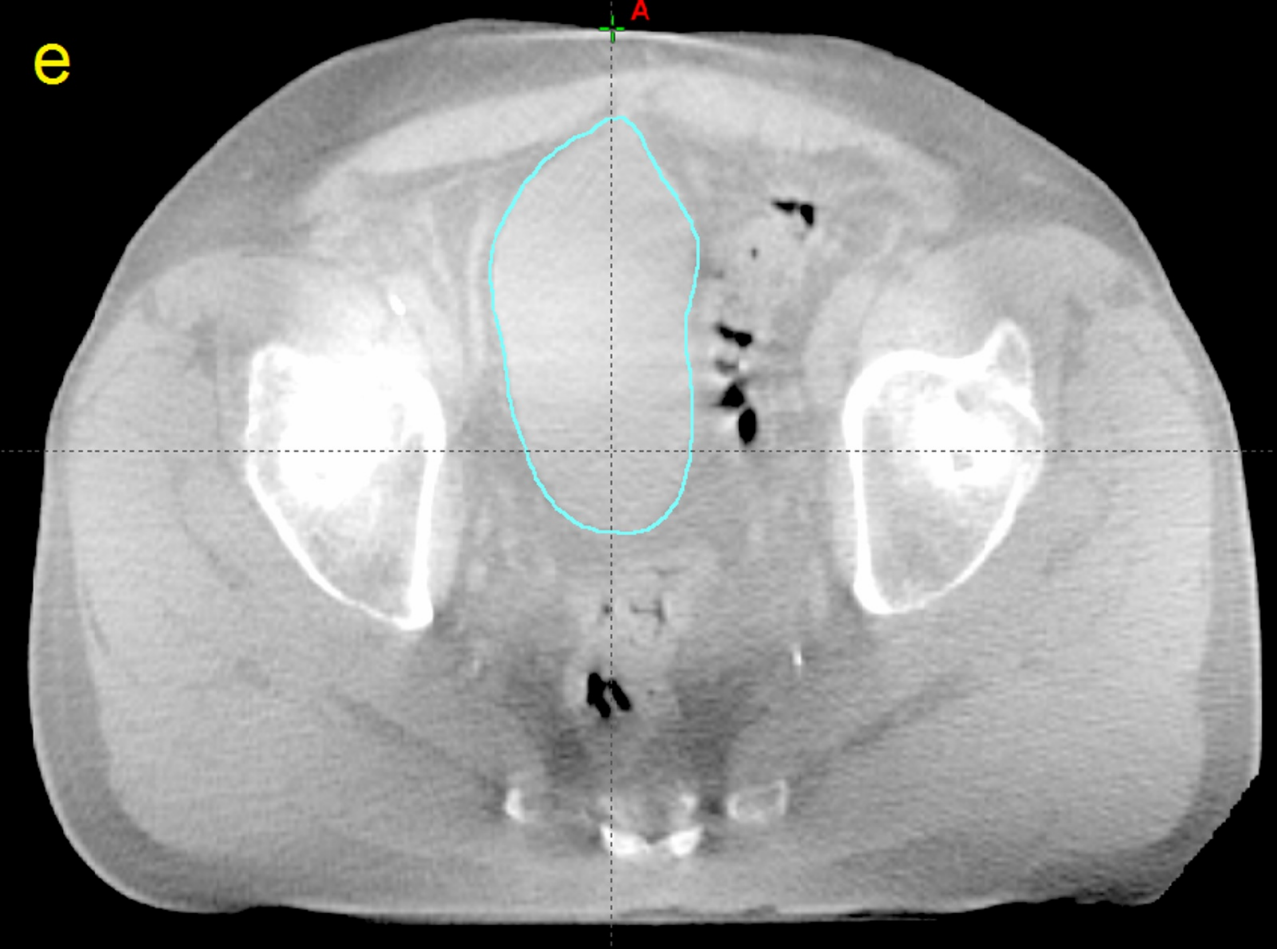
Computed tomographic (CT) image taken from patient P12 e) The cone beam computed tomography slice after contouring (teal).
